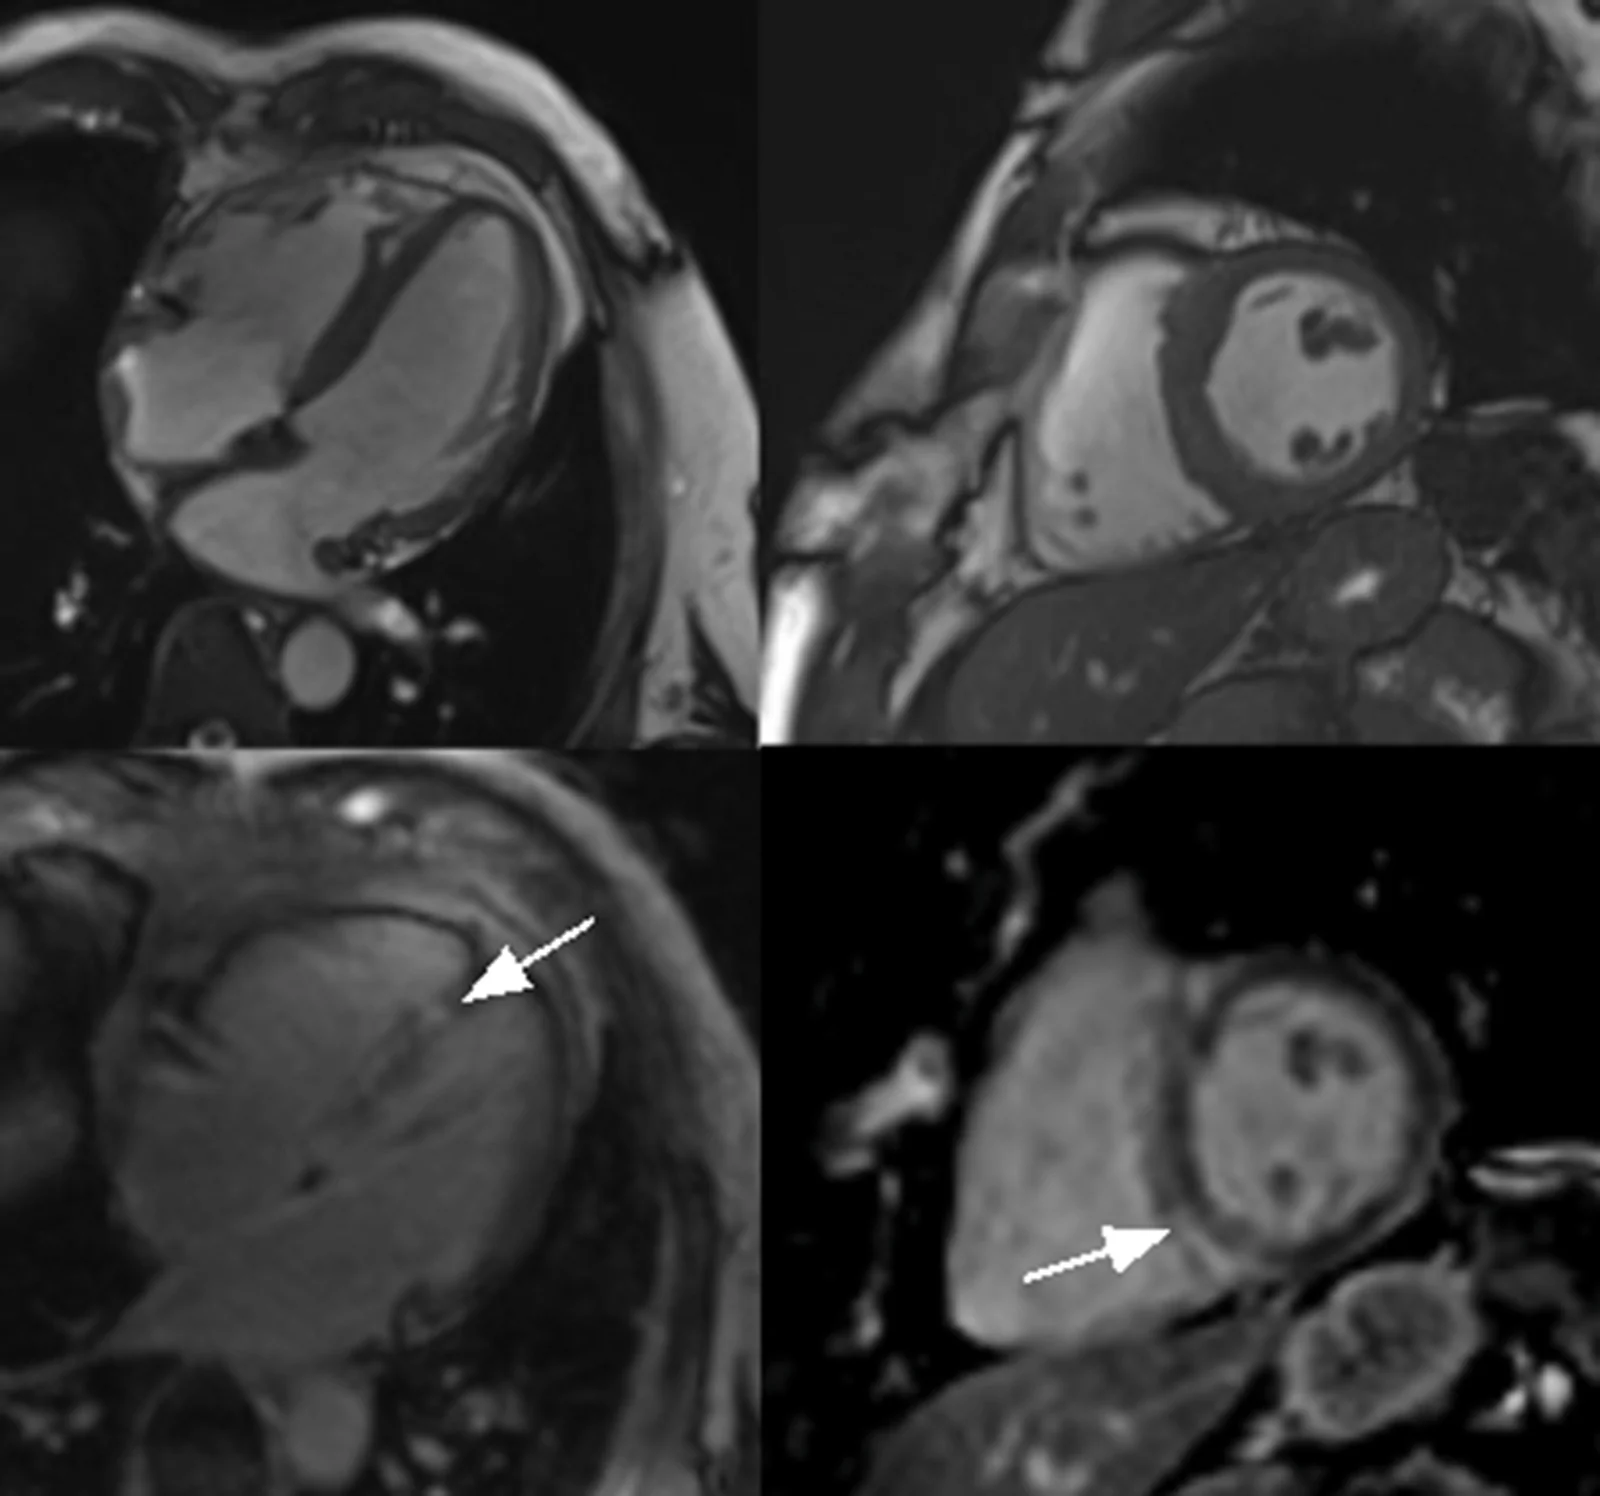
Cardiac Magnetic Resonance Imaging Cine imaging and late gadolinium enhancement sequences demonstrate increased interventricular septal thickness and diffuse, patchy, nonischemic myocardial enhancement, predominantly involving the intramyocardial and septal regions (white arrows), consistent with cardiac amyloidosis.
